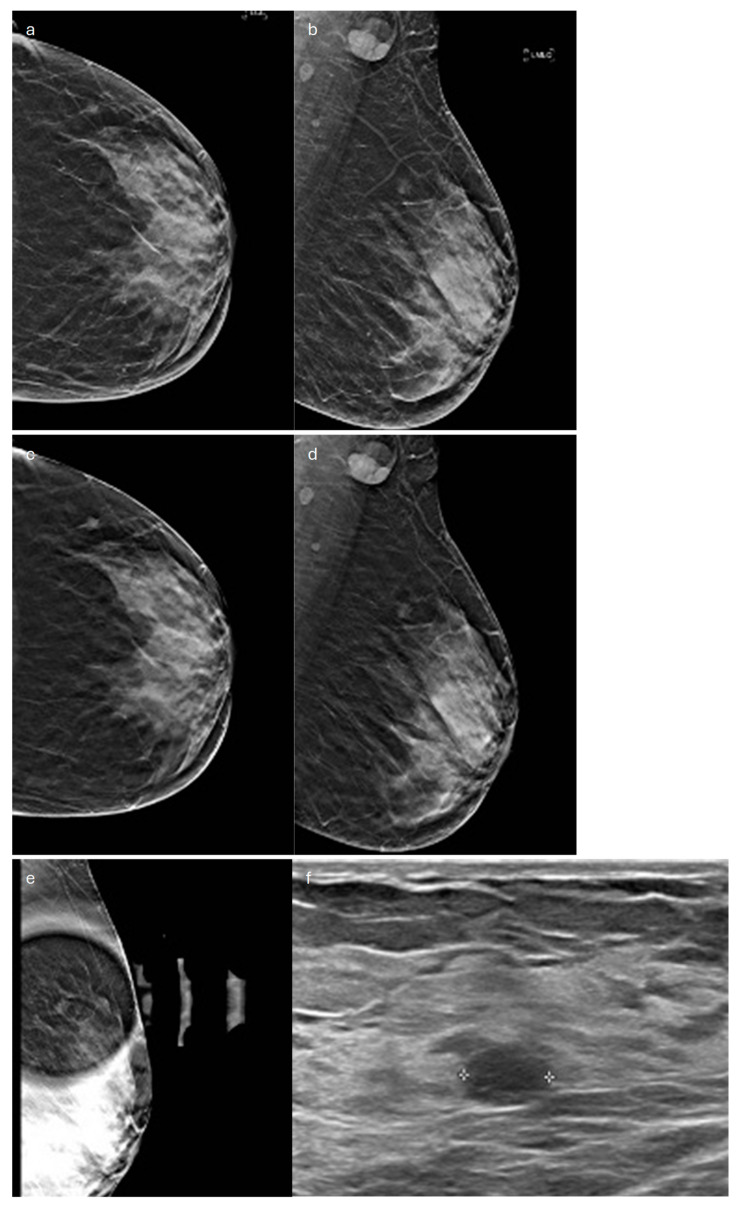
Medullary carcinoma in a 60-year-old patient. (a) Cranio-caudal and (b) medio-lateral oblique 2D synthetic mammograms, (c) cranio-caudal DBT image, (d) medio-lateral oblique DBT image and (e) spot compression view of the left breast showing an oval-shaped opacity in the upper-outer quadrant (f) corresponding to an oval-shaped homogeneous hypoechoic mass on ultrasound.
